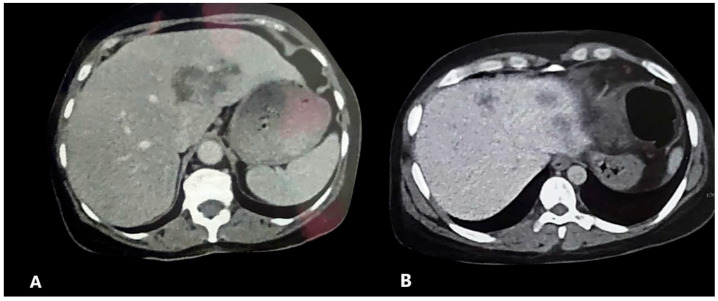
Pattern of different hypointense hepatic focal lesions by contrast-enhanced computed tomography in two patients (A,B) at enrollment in the study.
